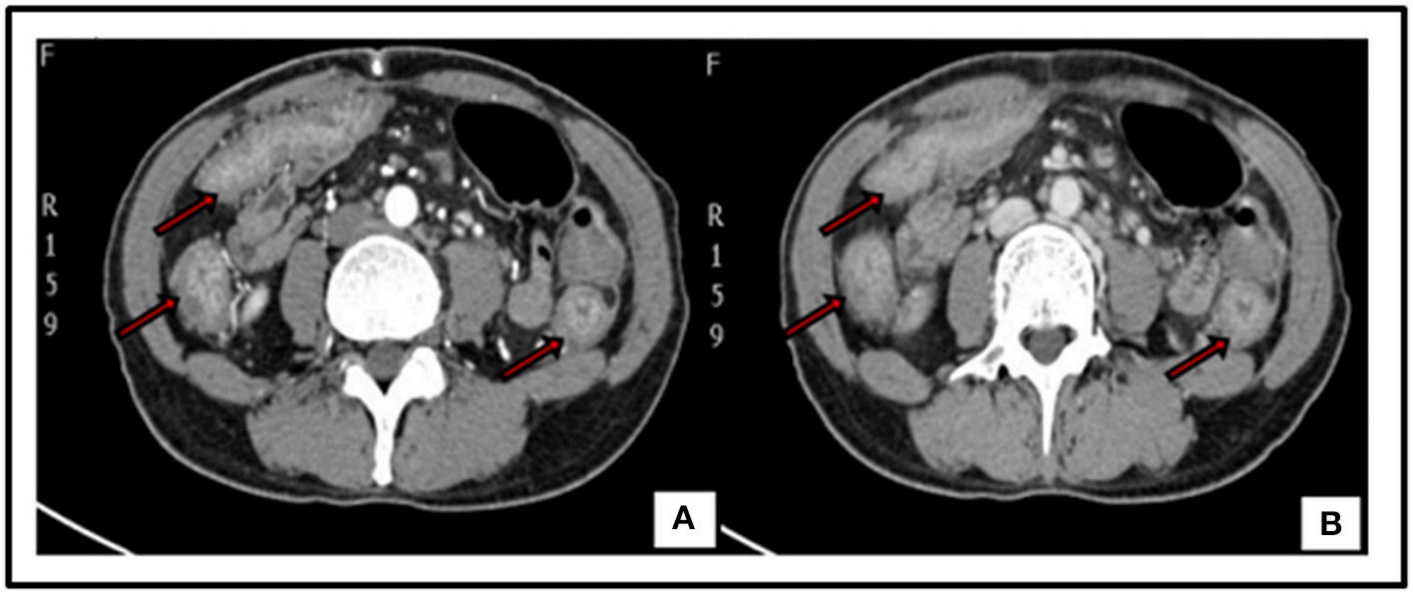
Enhanced-CT of abdomen: diffusible thickening mucosa of ascending, transverse and descending colon with coarse rims, uneven enhancement in artery phase (Red arrow) (A). Image of Venous phase (Red arrow) (B). Multiple lymph nodes could be seen in mesenteric and posterior-peritoneum areas.
